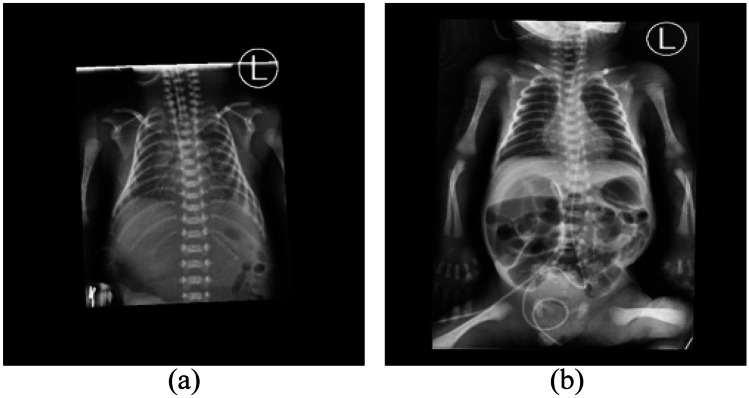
a. The chest radiograph taken at 0 h postnatal age in a preterm infant and treated with surfactant immediately b. The chest radiograph of the same infant taken at 47 h postnatal age after treatment with surfactant showed improving lung aeration
